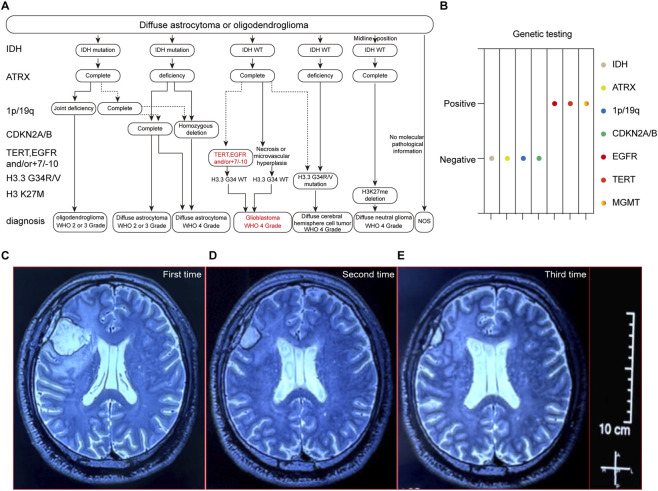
Clinical efficacy of LWF in glioma patients with high EGFR expression. (A) The Diagnosis and Treatment Guidelines for Gliomas (2022 Edition). (B) Genetic testing results. (C) Brain MRI images of the patient before treatment with LWF. (D) Brain MRI images of the patient after 2 months of LWF treatment. (E) Brain MRI images of the patient after 4 months of LWF treatment. The three magnetic resonance imaging test result maps are on the same scale.
